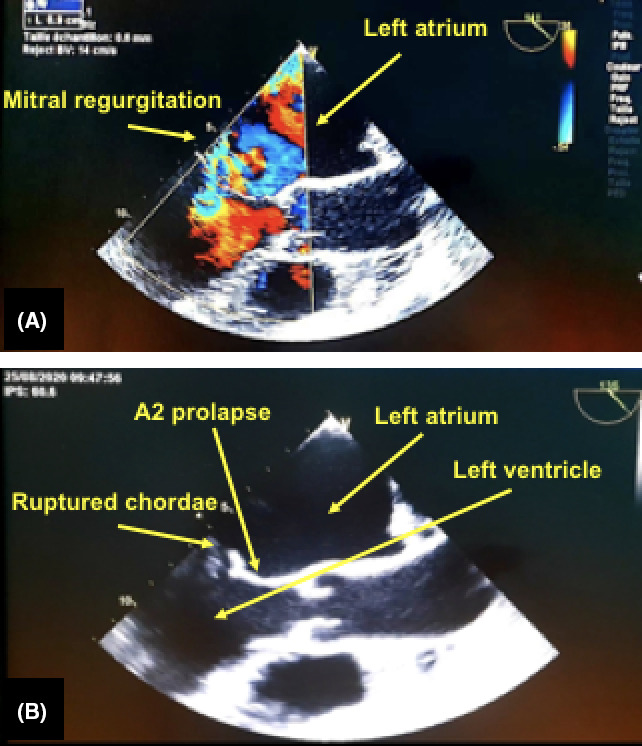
A transesophageal echocardiography showing a severe mitral regurgitation (A) by prolapse of the large mitral valve (A2 prolapse) and rupture of the chordae (B)
